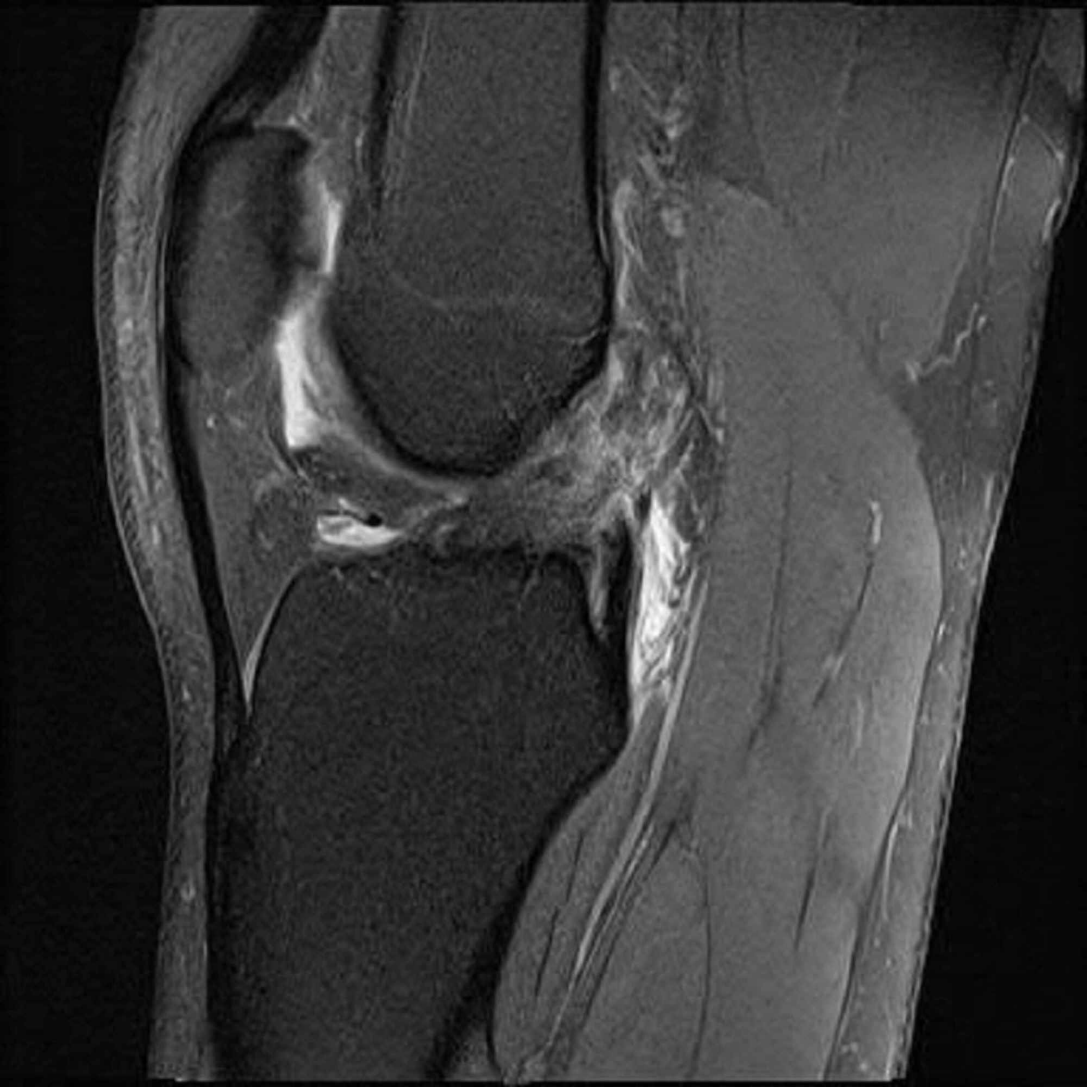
Magnetic resonance imaging (MRI) confirming an anterior cruciate ligament (ACL) tear with associated bone contusions in Patient A
